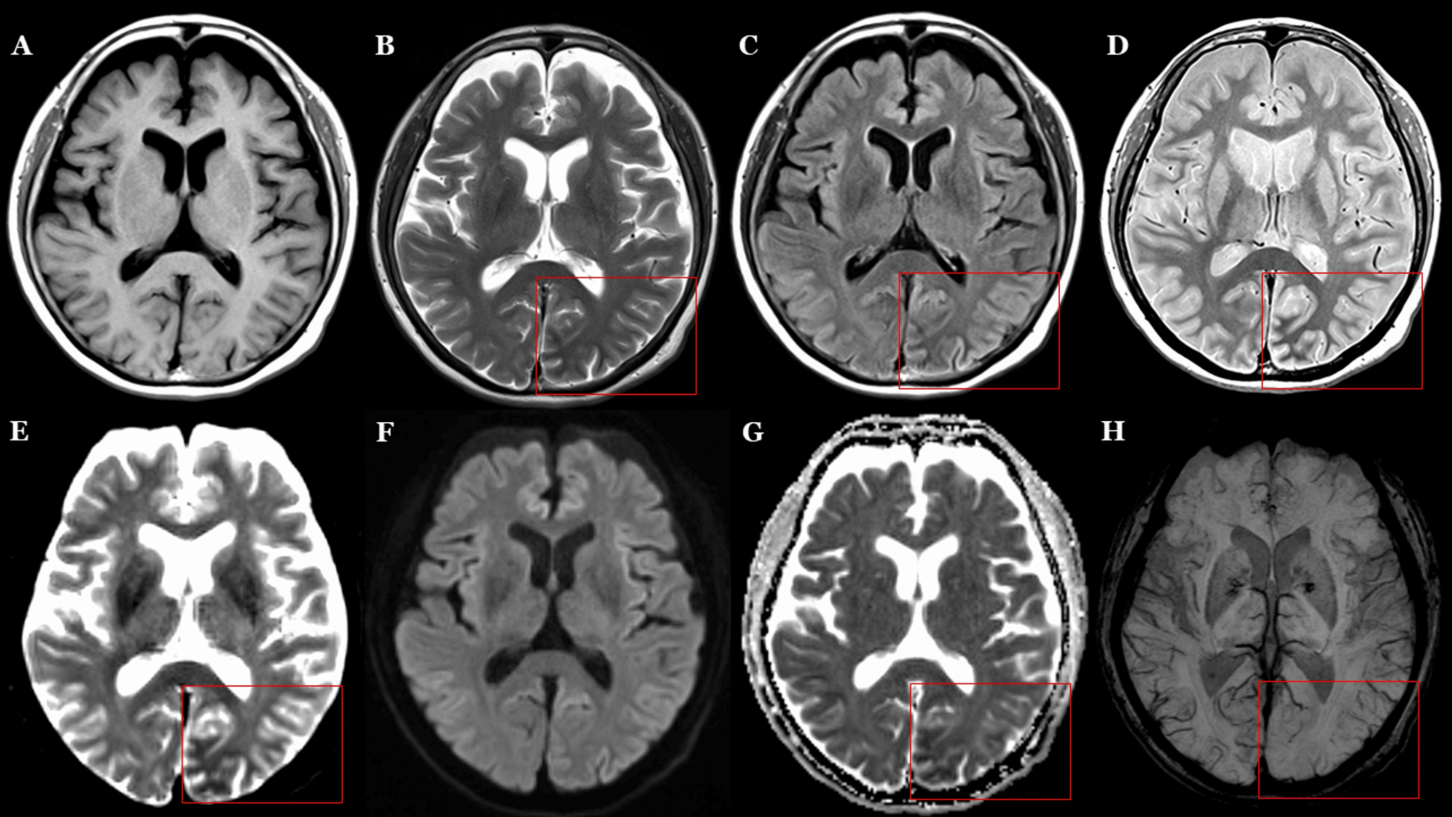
Head MRI on day 2 of admission (day 7 of right homonymous hemianopia onset) shows an area of abnormal signal (red frames) No abnormal findings are observed on T1-weighted imaging (A). Subcortical hypointensity in the left occipital lobe is observed on T2-weighted imaging (B), FLAIR (C), proton density weighted imaging (D), b=0 (E), and ADC map (G). No abnormalities are noted on DWI with a b-value of 1000s/mm² (F). On SWI (H), decreased visibility of veins in the left occipital lobe is noted, which may reflect increased regional perfusion. FLAIR: fluid-attenuated inversion recovery; ADC: apparent diffusion coefficient; DWI: diffusion-weighted imaging; SWI: susceptibility-weighted imaging
